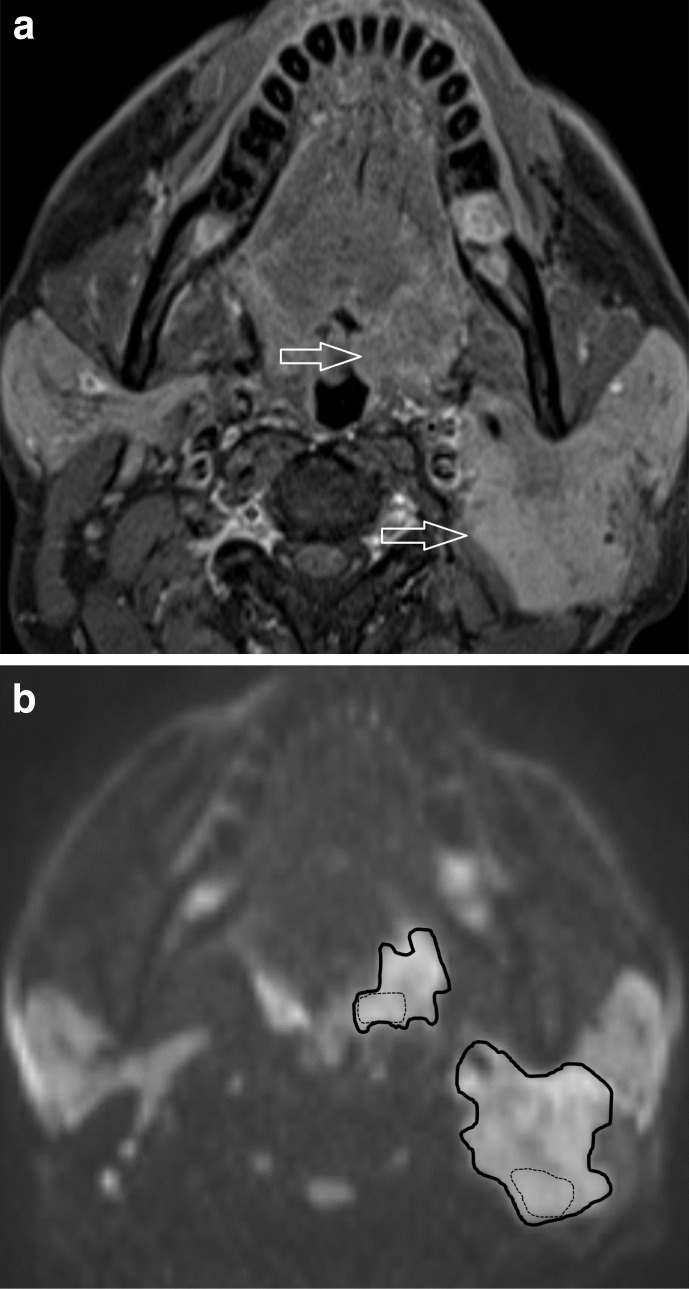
A HPV negative oropharyngeal cancer patient with a left palatine tonsillar cancer and left level two necrotic lymph node. (a) T1w post gadolinium axial image demonstrates the left palatine tonsillar tumour and the left level two lymph node (arrows). (b) *b* = 800 s/mm^2^ map from DW-MRI indicating aROIs as the continuous bold lines and rROIs as the dotted lines. DW-MRI, diffusion-weighted MRI; HPV, human papilloma virus; ROI, region of interest.
